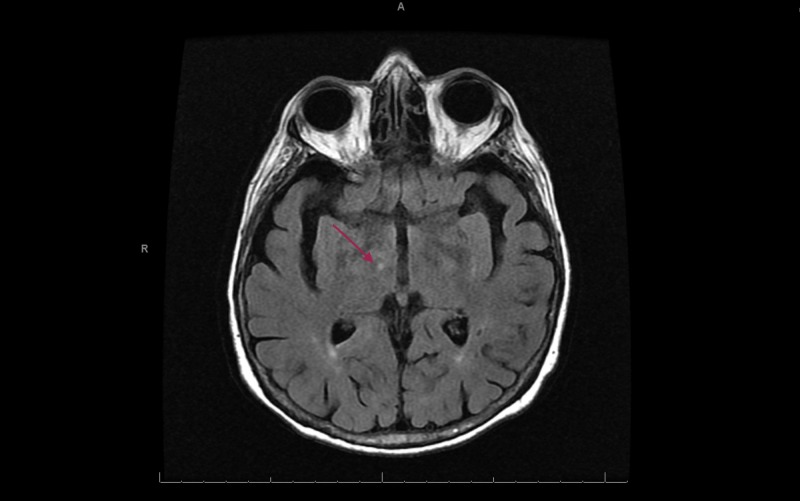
MRI brain axial T2 of acute to subacute right thalamic lacunar infarct.
